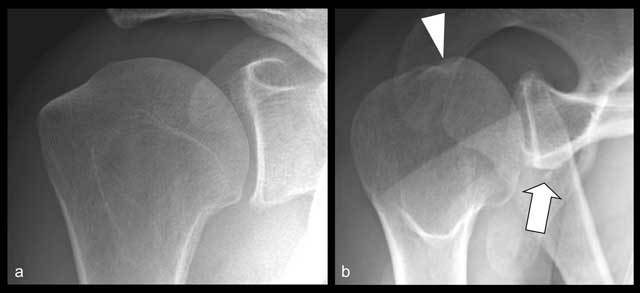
Hill-Sachs and Bankart fractures on Garth view. (a) Frontal shoulder radiograph (a) showing no abnormality. Garth view (b) showing a postero-superior depression of humeral head (arrowhead) and fracture of antero-inferior margin of glenoid (arrow).
